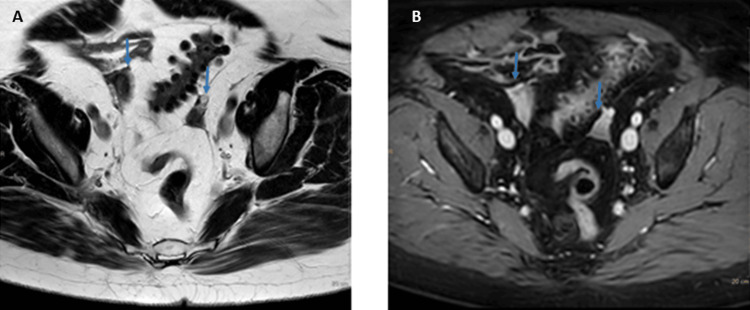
MRI showing both adnexa (blue arrows) in Case 2 Left (panel A): T2-weighted image showing the heterogeneous appearance of adnexa with the presence of a few millimetric cysts (<5 mm) and a central moderate hypointense signal intensity. Right (panel B): T1-weighted image (3d Dixon fs) after injection of Gadolinium. The solid components show enhancement.
